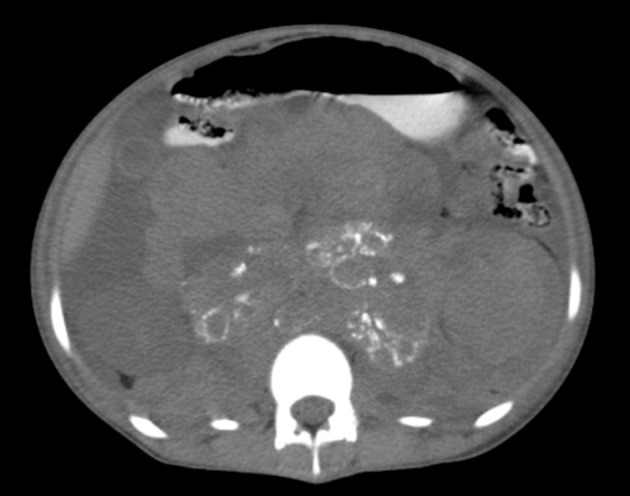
Abdominal CT scan of the patient with oral without IV contrast shows calcification of the tumoral mass
